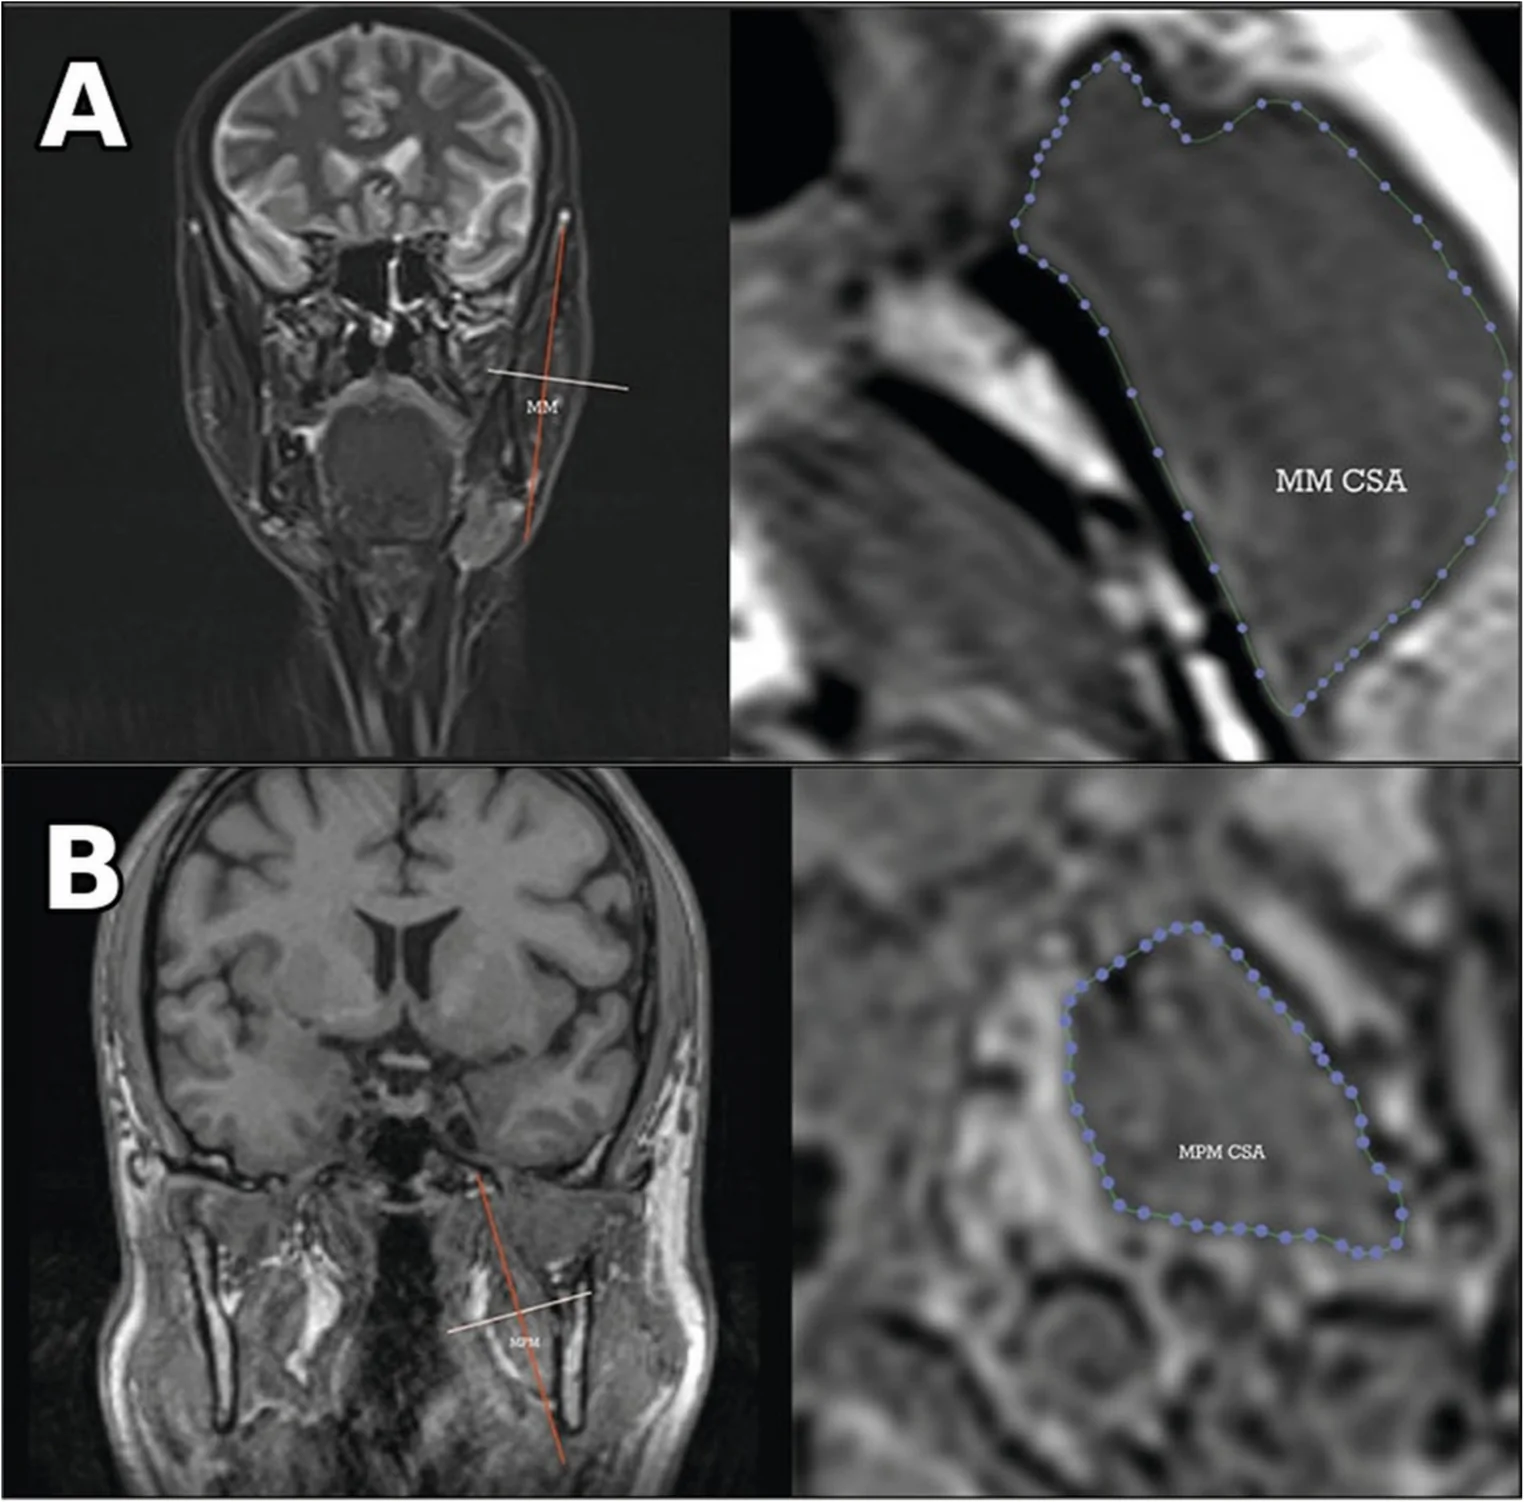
Determination of (A) the masseter and (B) medial pterygoid muscle’s long axis based on a coronal T2-weighted inversion recovery sequence and assessment of the corresponding cross-sectional area perpendicular to the muscle’s long axis on a transverse T1-weighted sequence
